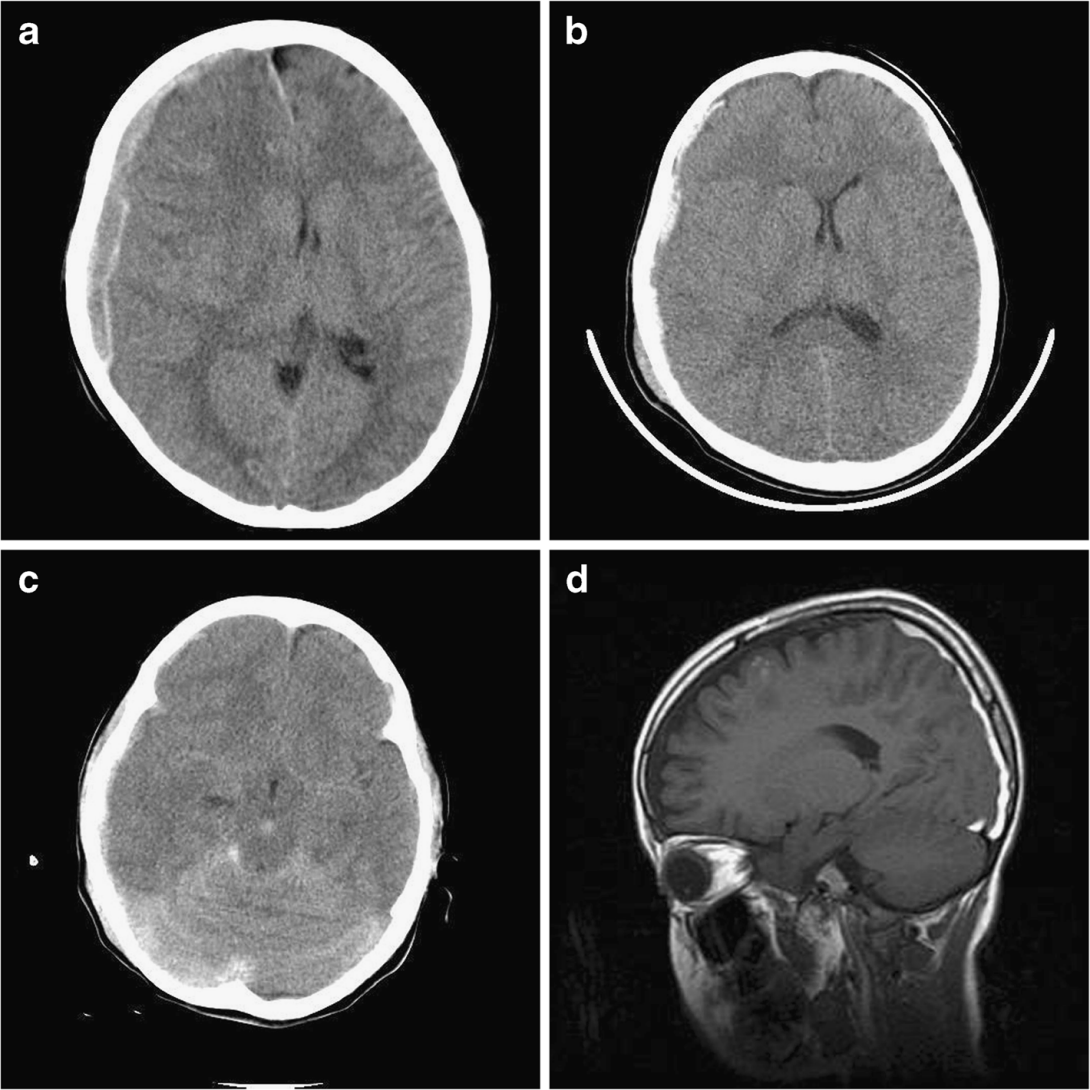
a: Initial computed tomography scan demonstrating mixed density of right hemispheric acute subdural hematoma with midline shift of more than 10mm. b: Computed tomography (CT) scan two hours after the accident showing marked reduction of the hematoma with more condensed density and improvement of the midline shift. c: CT scans six hours after the accident showing prominent subarachnoid hemorrhage and redistribution of the hematoma into the tentorial and interhemispheric subdural spaces. d: Sagittal T_1_-weighted magnetic resonance image, obtained two weeks after the accident, revealing a subacute-staged subdural hematoma with frontal contusion.
